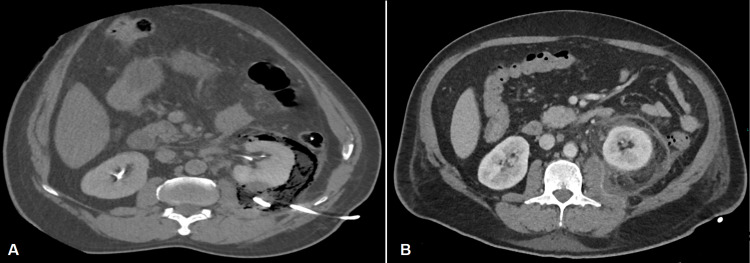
Enhanced abdominal CT axial slices at the left mid renal pole on the second (A) and eleventh (A) days of admission showing the progression of the perinephric collection after catheter drainage An accurately placed pigtail drainage catheter is seen (A) in the left posterior perinephric space at the lower margins of the subcapsular mixed collection and perinephric gaseous collection of type IIIB as per the Huang and Tseng classification. A follow-up image (B) acquired on day 10 post-drainage (i.e. day 11 post-admission) demonstrates persistent Gerota's fascia thickening with perinephric stranding and collection, however, without bubbly or linear streaks of gas.
